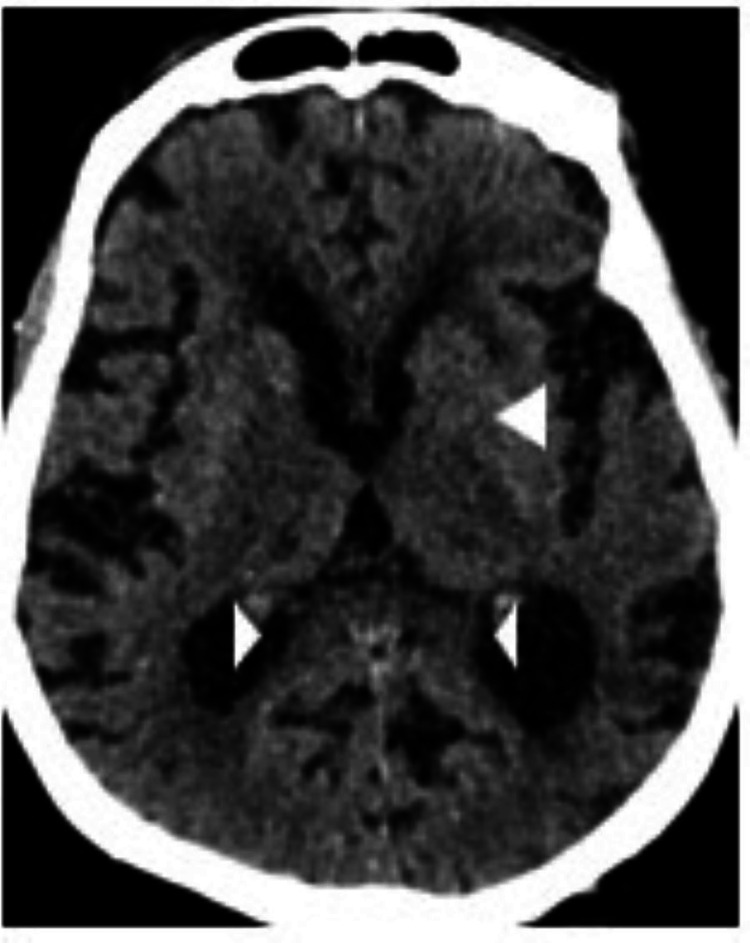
Brain CT of patient two The image shows bilateral thalamic and left external capsule chronic infarcts (top arrowhead) and age-related involutional changes noted with the widening of the sulci and dilatation of the ventricles (bottom arrowheads). There are periventricular hypodensities in keeping with chronic small vessel ischemic changes.
